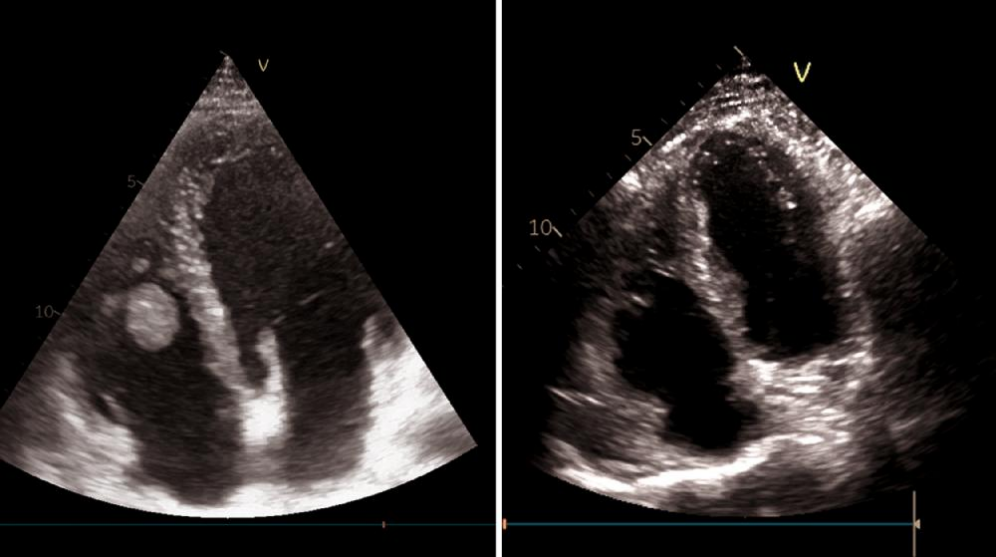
On the left: 2D TTE (apical four-chamber view) performed after PE diagnosis showing a large thrombotic mass in the right ventricle. On the right: 2D TTE (apical four-chamber view) performed after 5 months of target therapy. Thrombosis was not visible anymore.
